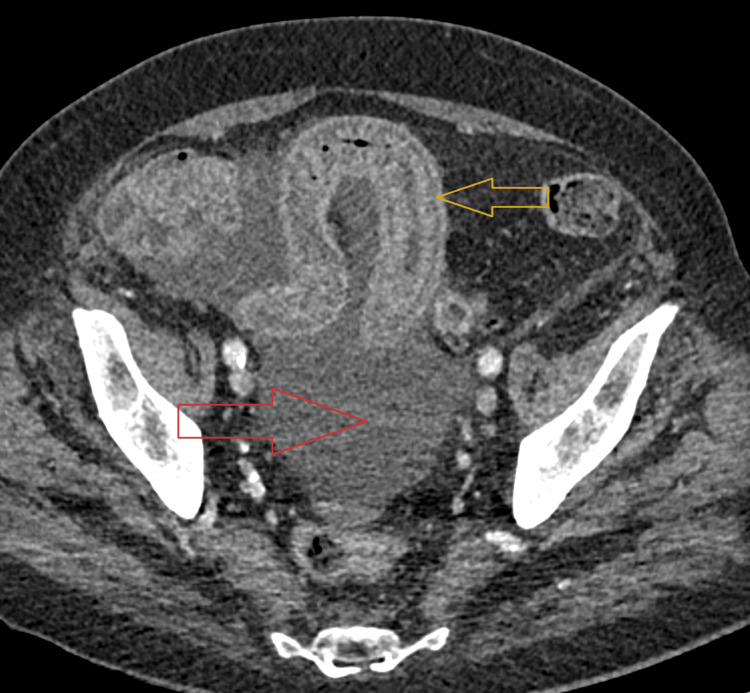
Axial contrast-enhanced CT image of the pelvis showing marked bowel wall edema (yellow arrow) with ascites (red arrow).
